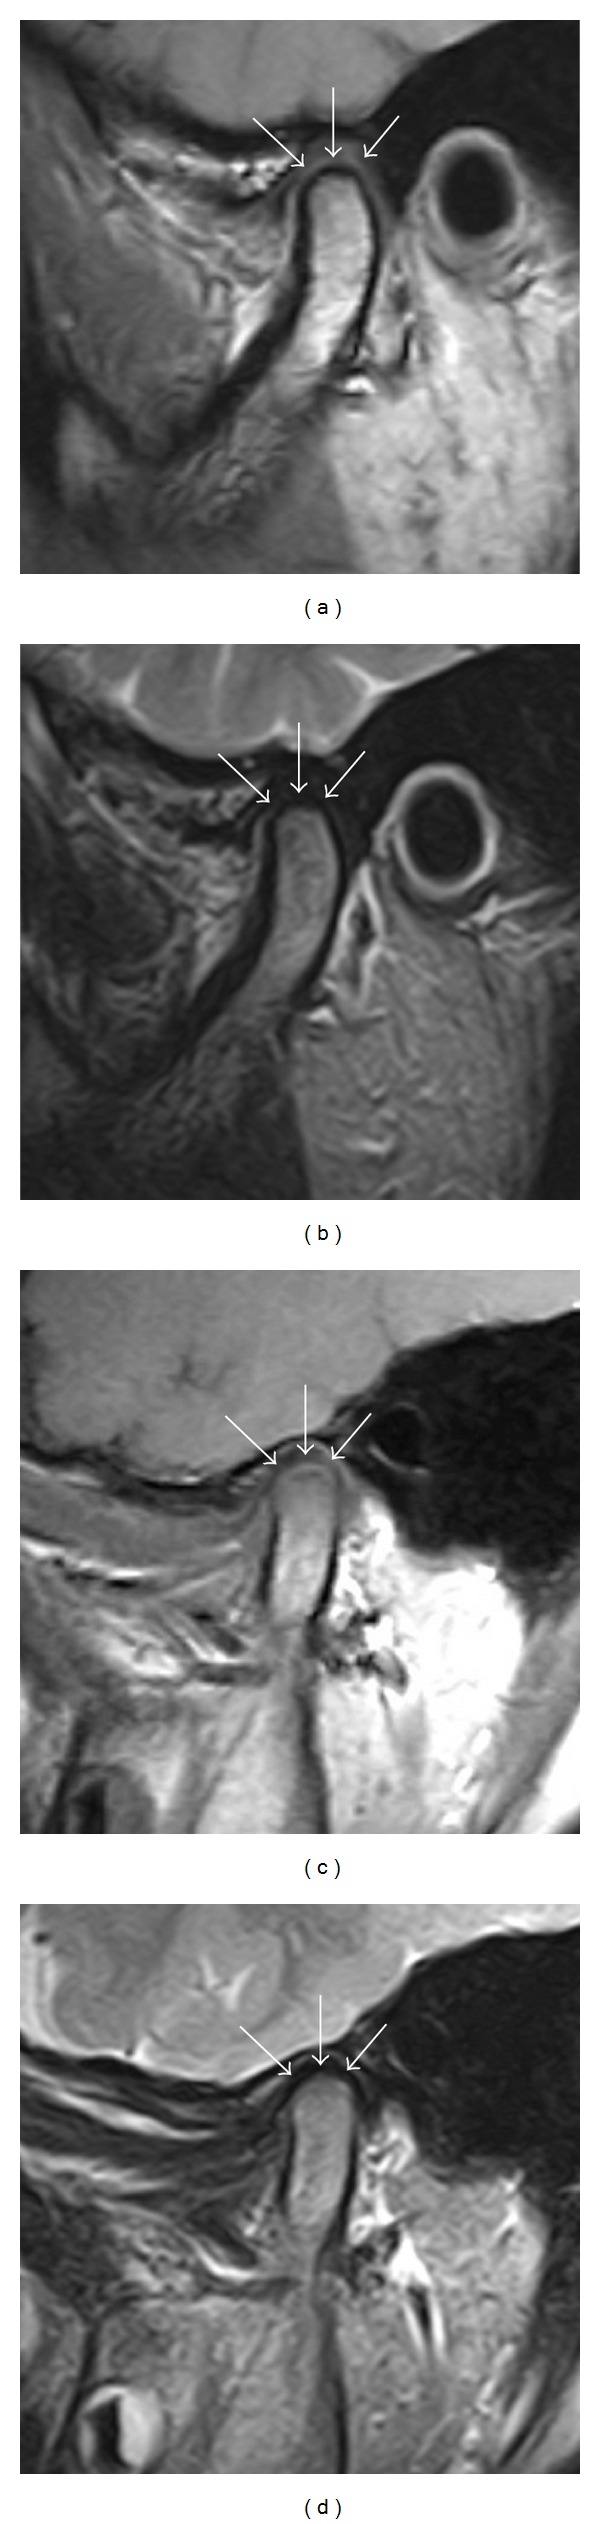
Differences in TMJ between normal adults (a, b) and schoolchildren (c, d) on MRI. (a) DCLS on the top of the mandibular condyles was undetectable (arrows) on fast spin-echo intermediate-weighted images of a healthy 34-year-old female. The voided signal line at the top of the right mandibular condyle was seen as a successive continuity. The imaging parameters used in the MR sequences included 1050 ms TR, 15 ms effective echo time, flip angle of 90°, a 15 × 15 cm field of view, a matrix of 160 × 288 pixels, 17 mm slab thickness, and 3 mm section thickness. (b) High-intensity stripes on the top of the mandibular condyles were undetectable (arrows) on fast spin-echo T2-weighted images of the same subject as Figure 1(a). The imaging parameters used in the MR sequences included 3500 ms TR, 108 ms effective echo time, flip angle of 90°, a 15 × 15 cm field of view, a matrix of 160 × 288 pixels, 17 mm slab thickness, and 3 mm section thickness. (c) DCLS on the top of the mandibular condyles (arrows) appeared as high-intensity stripes over a low-intensity area on fast spin-echo intermediate-weighted images of a healthy 8-year-old boy. The voided signal line at the top of the right mandibular condyle was not seen. The imaging parameters used in the MR sequences included 1050 ms TR, 15 ms effective echo time, flip angle of 90°, a 15 × 15 cm field of view, a matrix of 160 × 288 pixels, 17 mm slab thickness, and 3 mm section thickness. (d) High-intensity stripes (arrows) were found to coincide with DCLS on the top of the mandibular condyles; this was found using fast spin-echo T2-weighted images of the same subject as Figure 1(c). The imaging parameters used in the MR sequences included 3500 ms TR, 108 ms effective echo time, flip angle of 90°, a 15 × 15 cm field of view, a matrix of 160 × 288 pixels, 17 mm slab thickness, and 3 mm section thickness.
